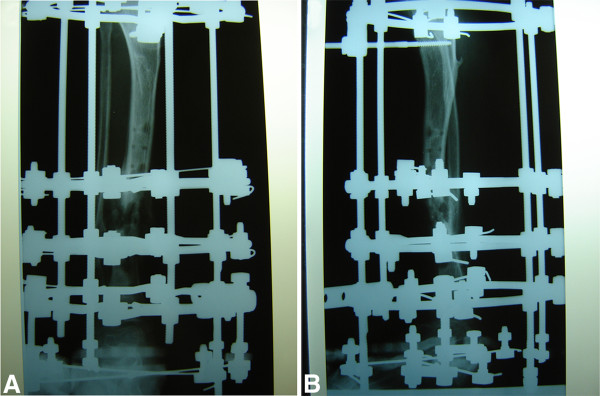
(a,b) Post-operative plain radiographs with the Ilizarov fixateur of the lower extremity (anterior-posterior and axial).
